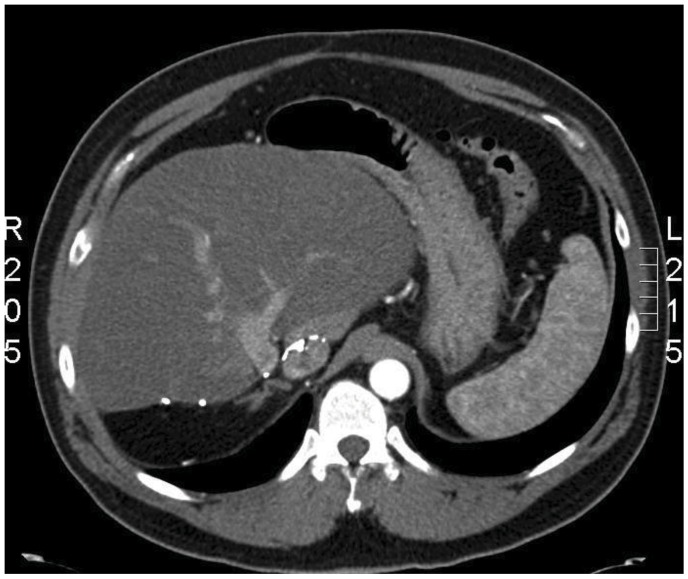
An enhanced CT scan of the abdomen 5 years following surgery demonstrates no evidence of recurrent disease in the liver.
